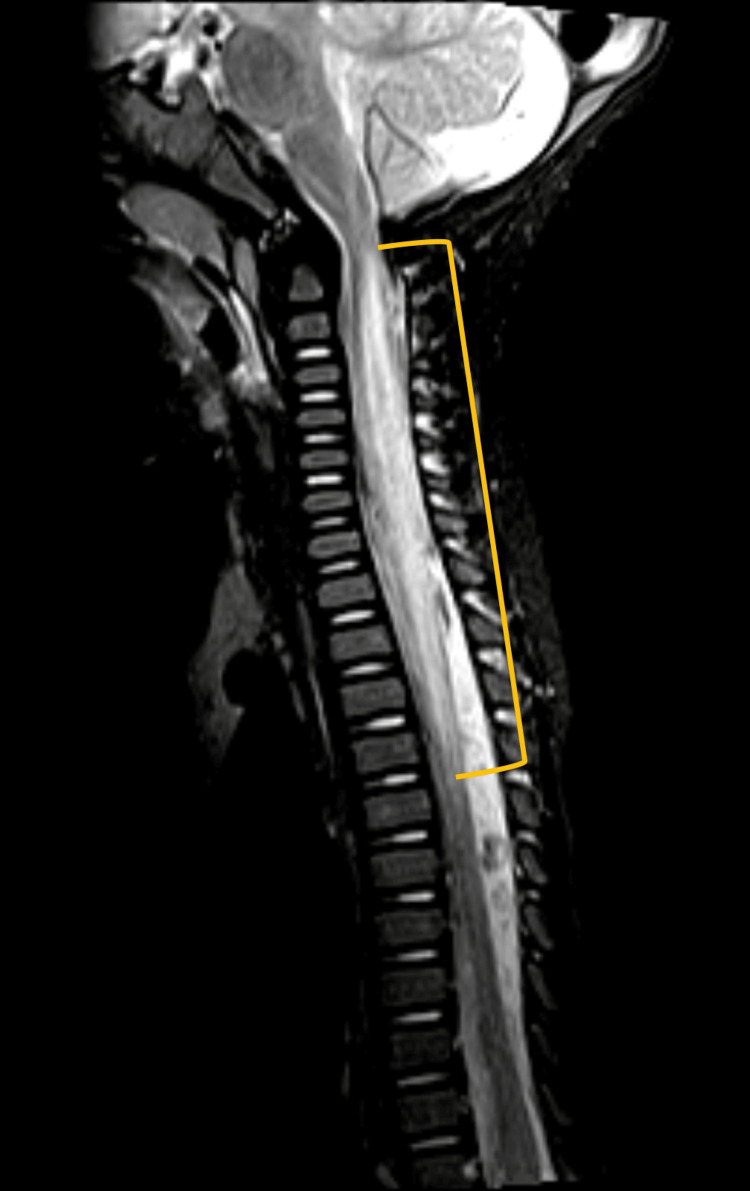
Magnetic resonance imaging of the spinal cord (T2/STIR sequence, sagittal plan) showing longitudinal extensive myelitis from the lower plane of C1 to D4-D5 (marked in yellow).
